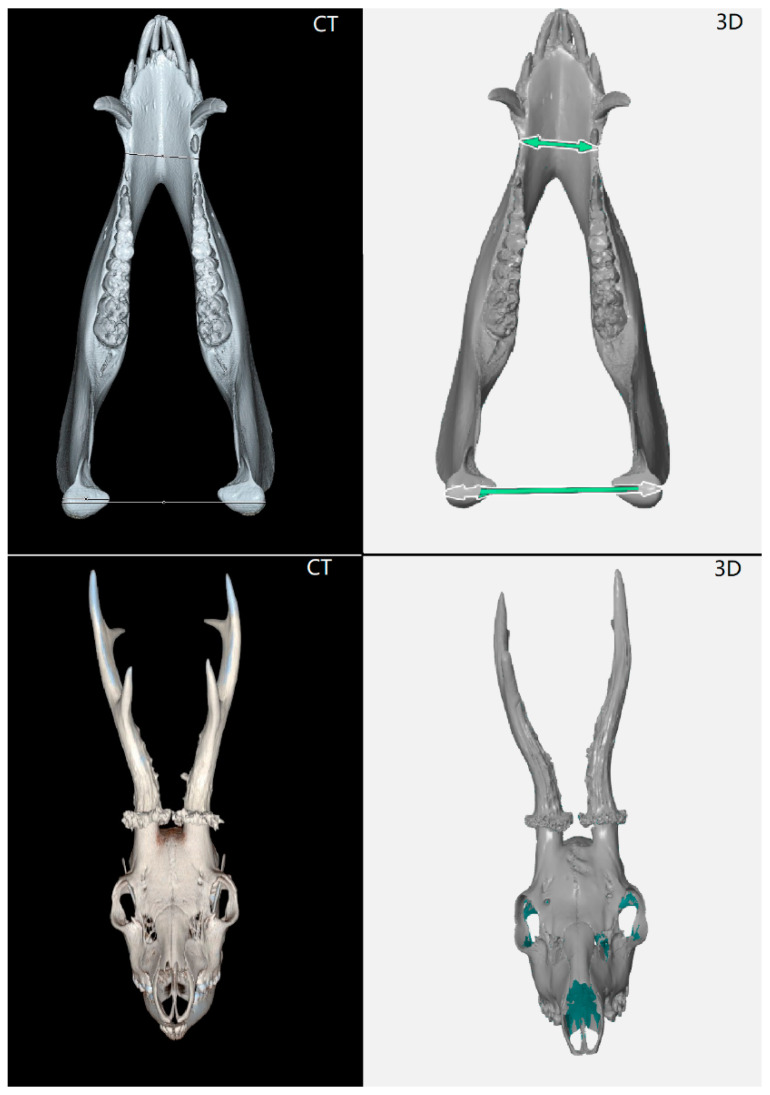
Comparison of imaging capabilities of a CT/3D scanner.
